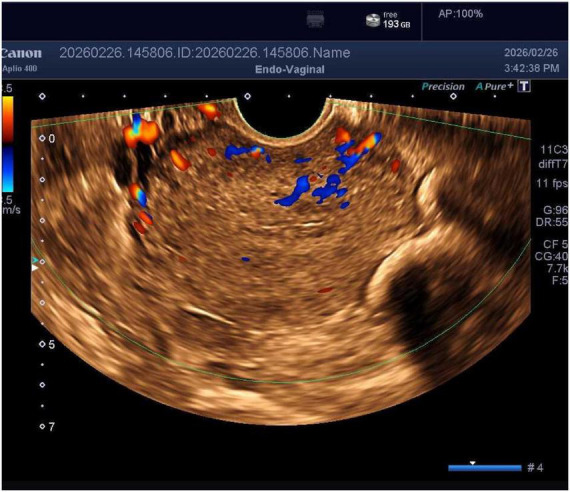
Postoperative follow-up transvaginal color Doppler ultrasound at 3 months demonstrating no residual intracavitary mass, with a normal endometrial echo pattern.
